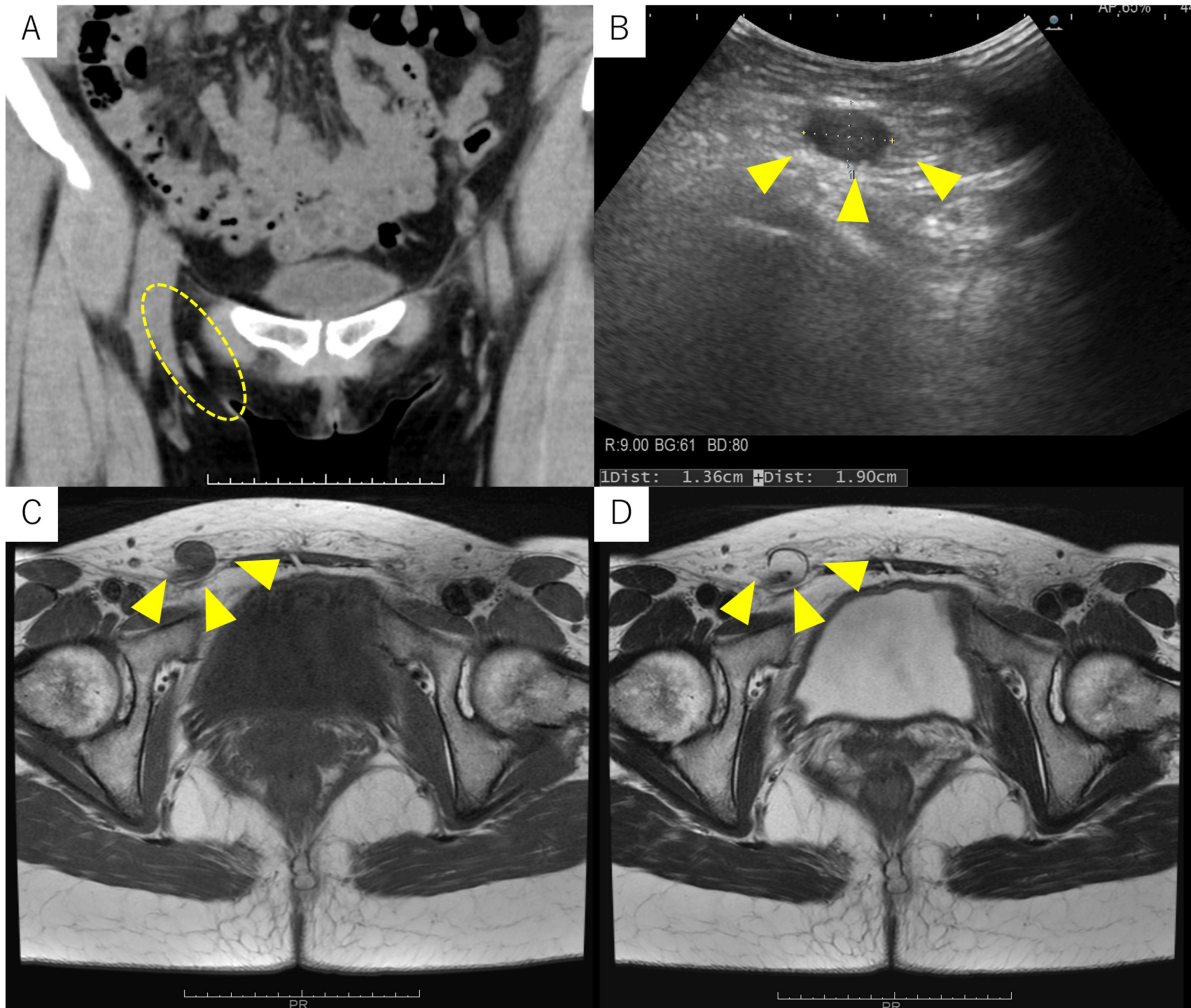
(A) CT imaging showed slight swelling of the right inguinal canal compared to the contralateral side (yellow dotted oval), raising the possibility of inguinal endometriosis. (B) Ultrasonography of the right inguinal region revealed a 1.36 x 1.90 cm subcutaneous mass (yellow arrowheads). (C) T1-weighted MRI revealed a structure exhibiting intermediate to low signal intensity within the right inguinal canal (yellow arrowheads). (D) T2-weighted MRI revealed a structure exhibiting high signal intensity within the right inguinal canal (yellow arrowheads). These findings raised suspicion for endometriosis of the right inguinal region.
